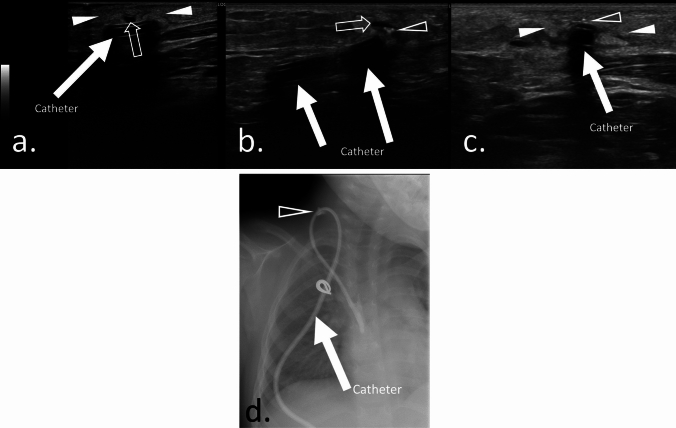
Case 1: Ultrasonographic findings in a 3.7-year-old girl with erythema and unplanned CVC extraction. The patient was diagnosed with complications within subcutaneous tissue due to catheter fracture at the tunneled area. The onset of symptoms was 45 days after catheter placement. The catheter was extracted 1 day after symptom onset. a. Ultrasonography showing a highly echoic area (solid arrowheads) around the catheter. Fluid collection around the catheter is also evident (vacant arrow). b. Ultrasonography showing fluid collection (vacant arrow) and highly echoic foci (vacant arrowhead) around the adjacent catheter. c. Hyperechoic foci (vacant arrowhead) and a highly echoic area (solid arrowheads) adjacent to the catheter were detected. d. Contrast study via catheter showing leakage of contrast medium (vacant arrowhead) from the catheter.
